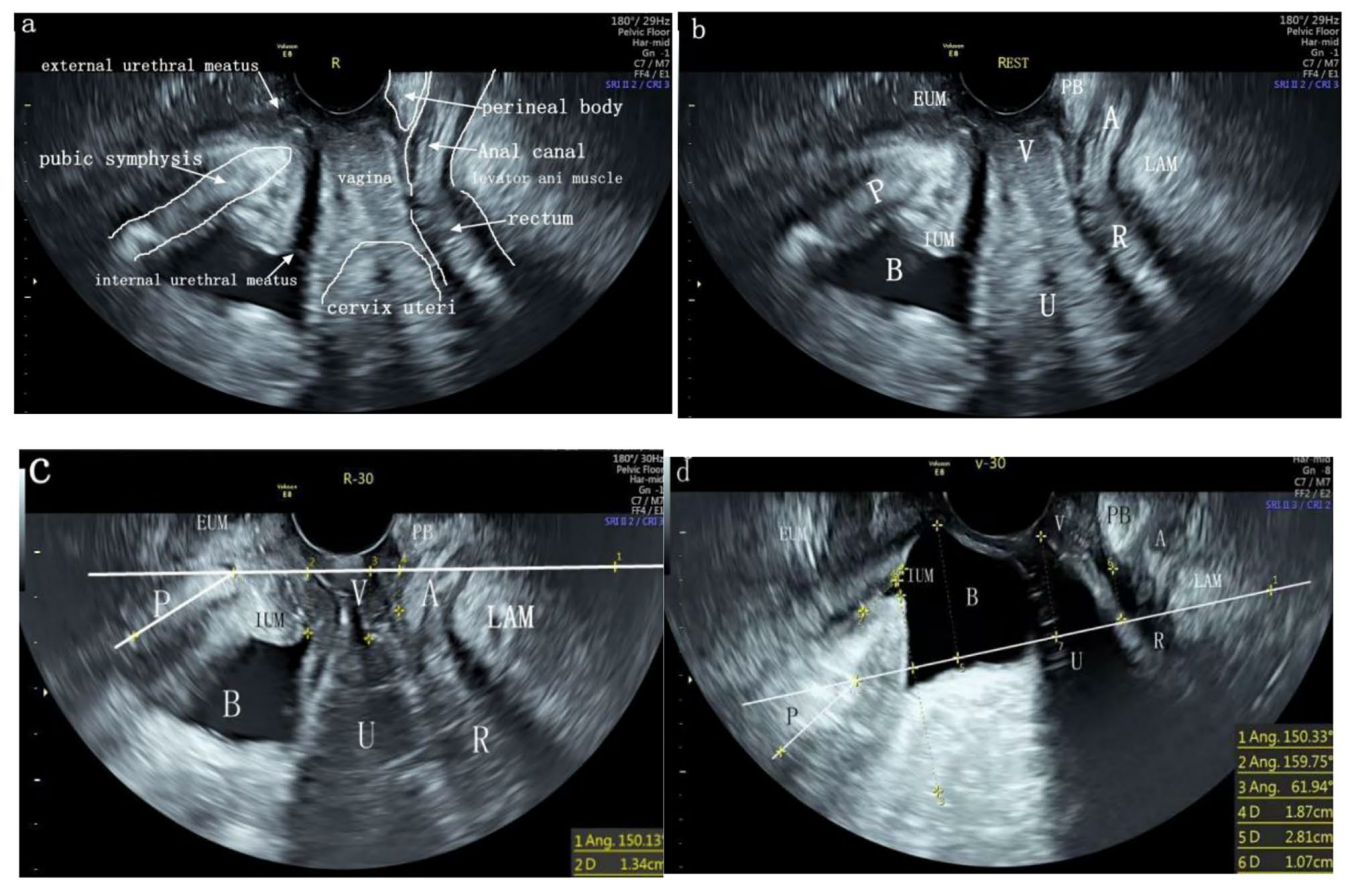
Images of the standard ultrasound plane of the pelvic floor organ. The distances between the organs and the 30° reference line of the ultrasound were measured when the women were at rest and exerting the maximum Valsalva breath-holding force (c and d). Pubic symphysis (P), bladder (B), vagina (V), cervix uteri (U), rectum (R), anal canal (A), perineal body (PB), internal urethral meatus (IUM), external urethral meatus (EUM), levator ani muscle (LAM)
